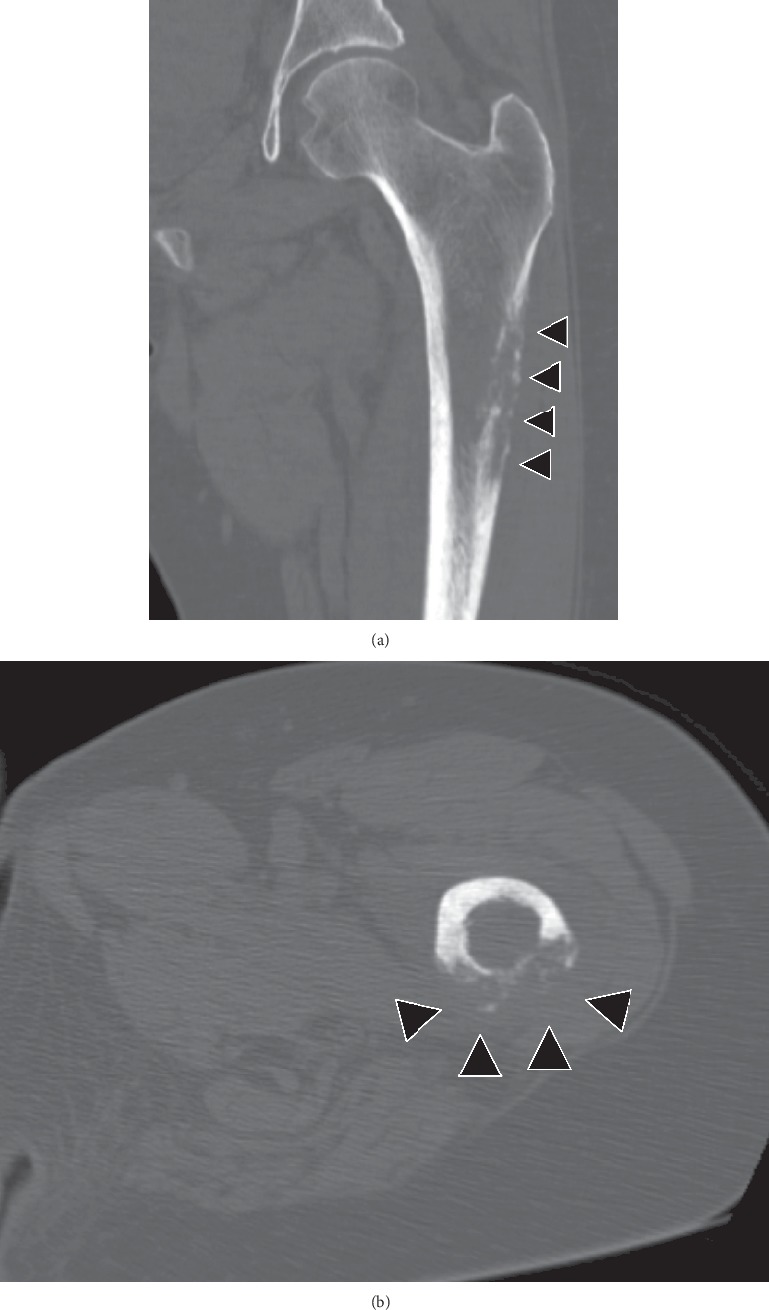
Computed tomography at first visit showing a purely osteolytic lesion on the left femur (arrowhead). (a) Coronal view and (b) axial view.
